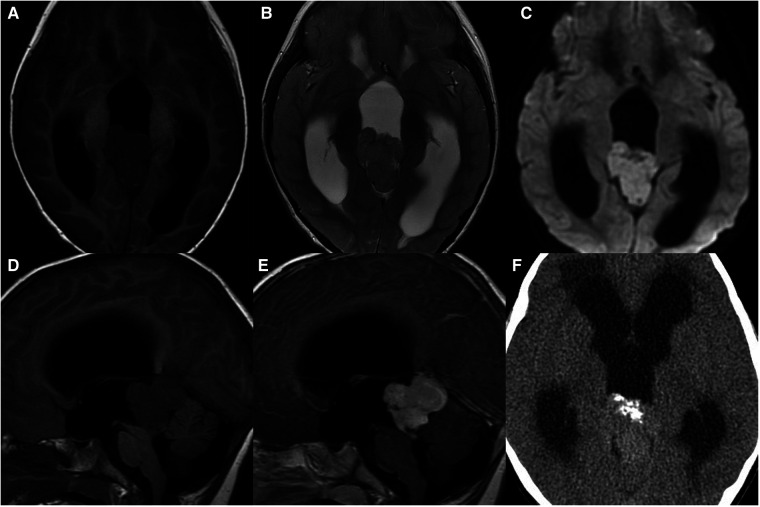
4-year-old boy with a pineoblastoma: axial T1WI (A), T2WI (B), and DWI (C), sagittal T1WI and post-contrast T1WI (D,E) show a lobulated mass with restricted diffusion, enhancement, and hydrocephalus; axial unenhanced CT (F) shows exploded calcification.
